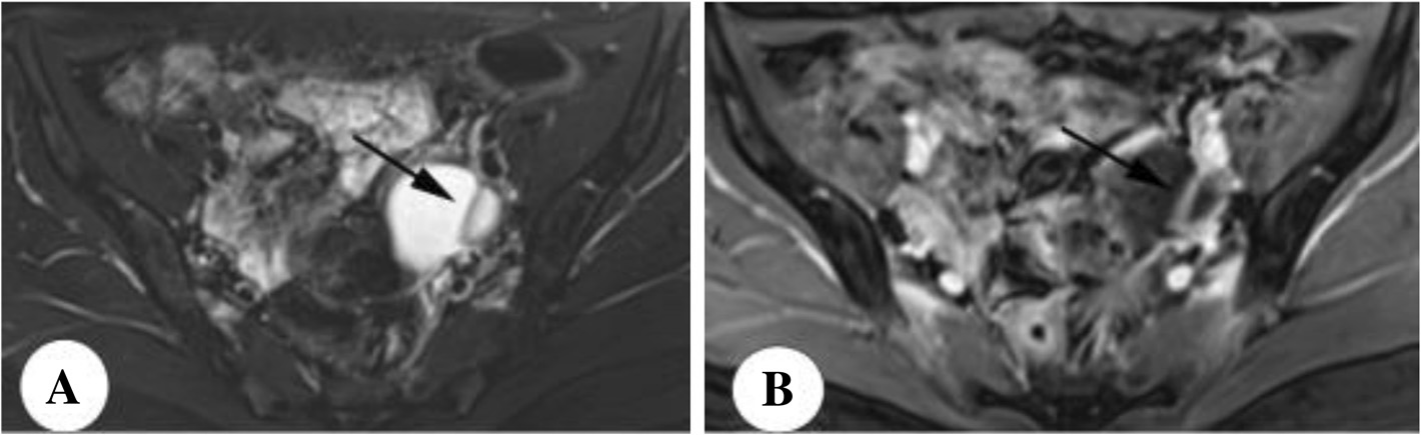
Sertoli-Leydig cell tumor in a 25-year-old woman with irregular menses. Axial T2-weighted image with fat saturation (A) shows a bilocular cystic mass with thickened septa and wall (arrow), which are intensely enhanced (B).
